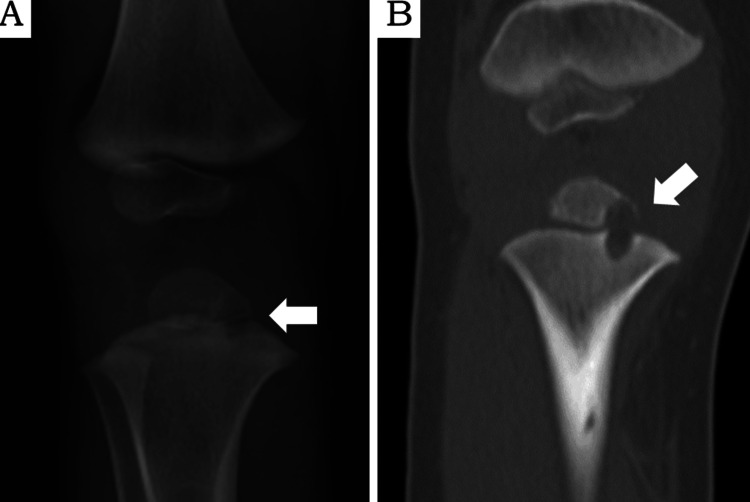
Radiographic images obtained at admission A: Plain radiograph of the right tibia showing a cystic lesion (arrow). B: Coronal computed tomography showing an osteolytic lesion in the metaphysis of the right tibia, extending to the physis and epiphysis.
